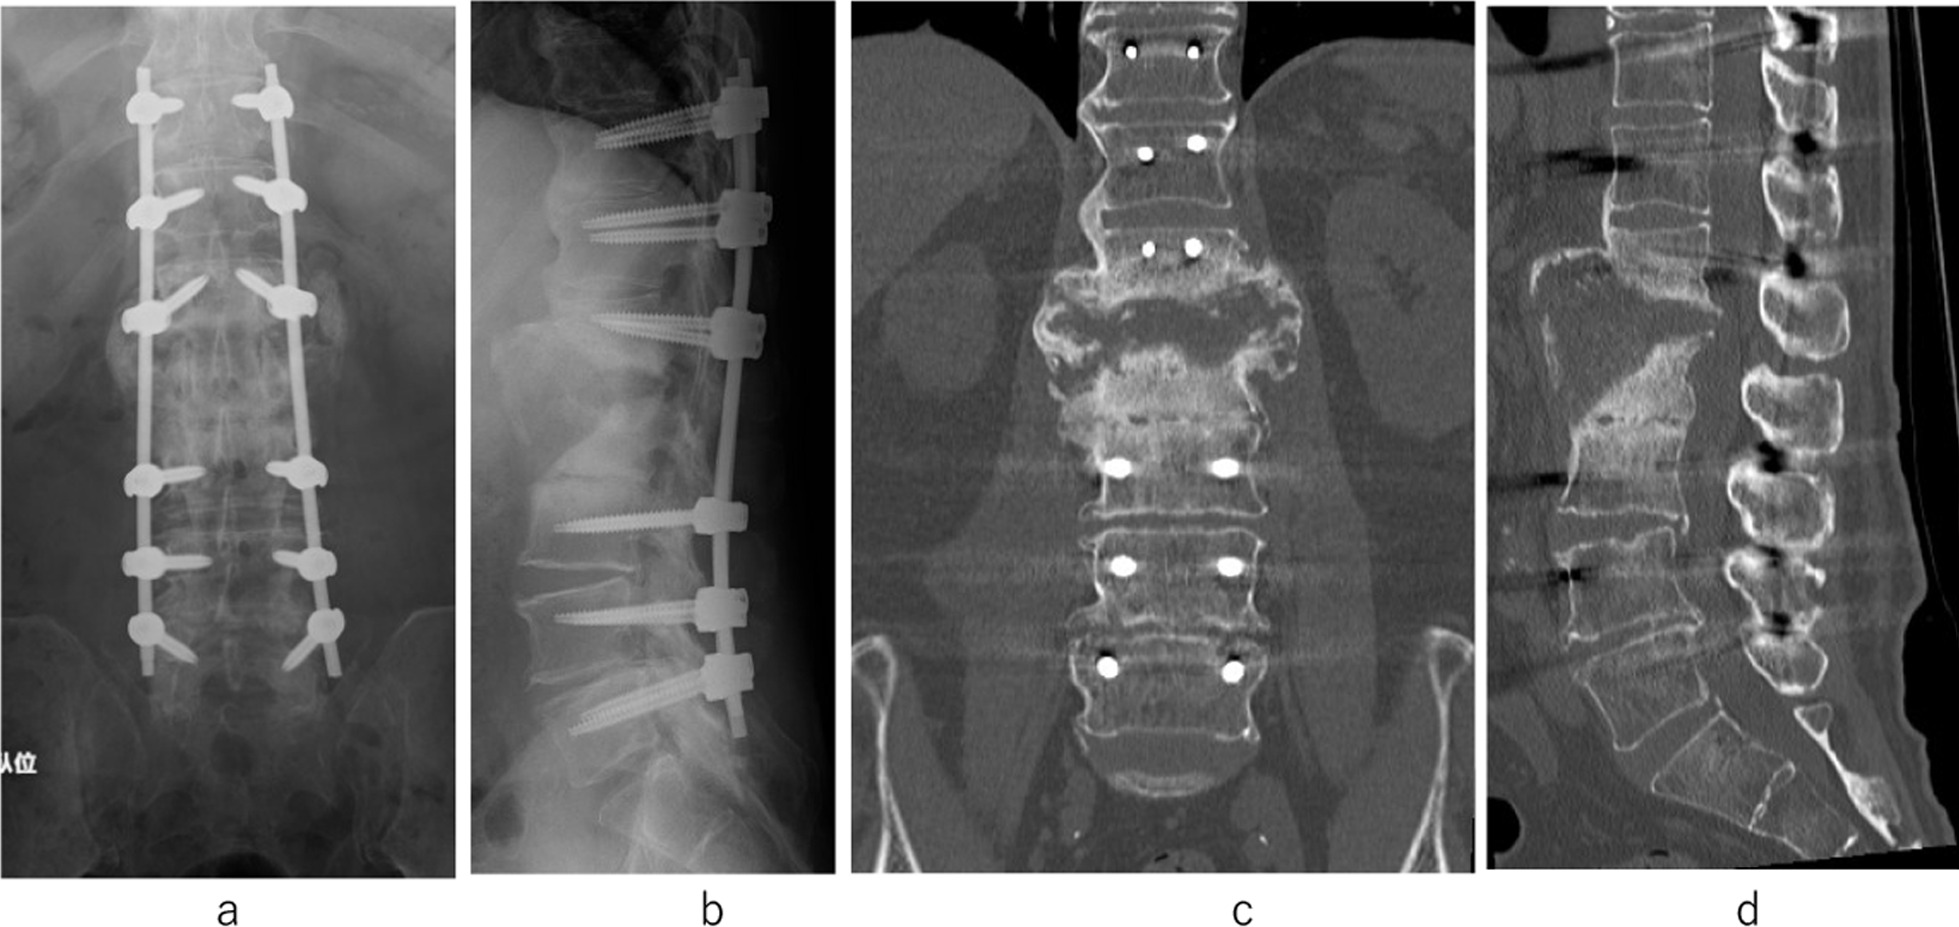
Plain X-ray imaging and computed tomography showing posterior fixation with percutaneous pedicle screws inserted in T11, T12, L1, L3, L4, and L5 vertebrae (a–d)
